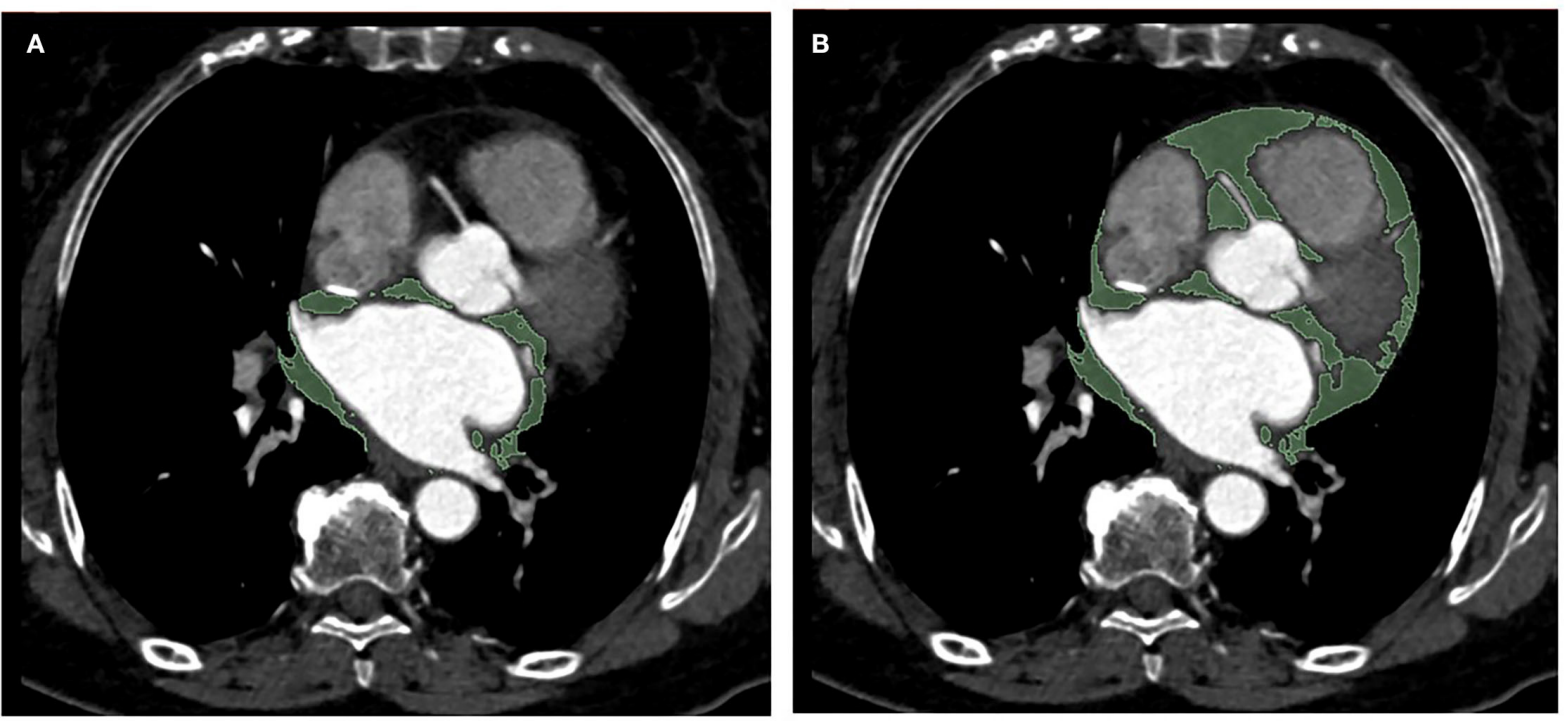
Representative example of periatrial EAT (A) and total EAT (B) measurement in a single axial CT image in a patient (area in green depict traced EAT). EAT, epicardial adipose tissue.
